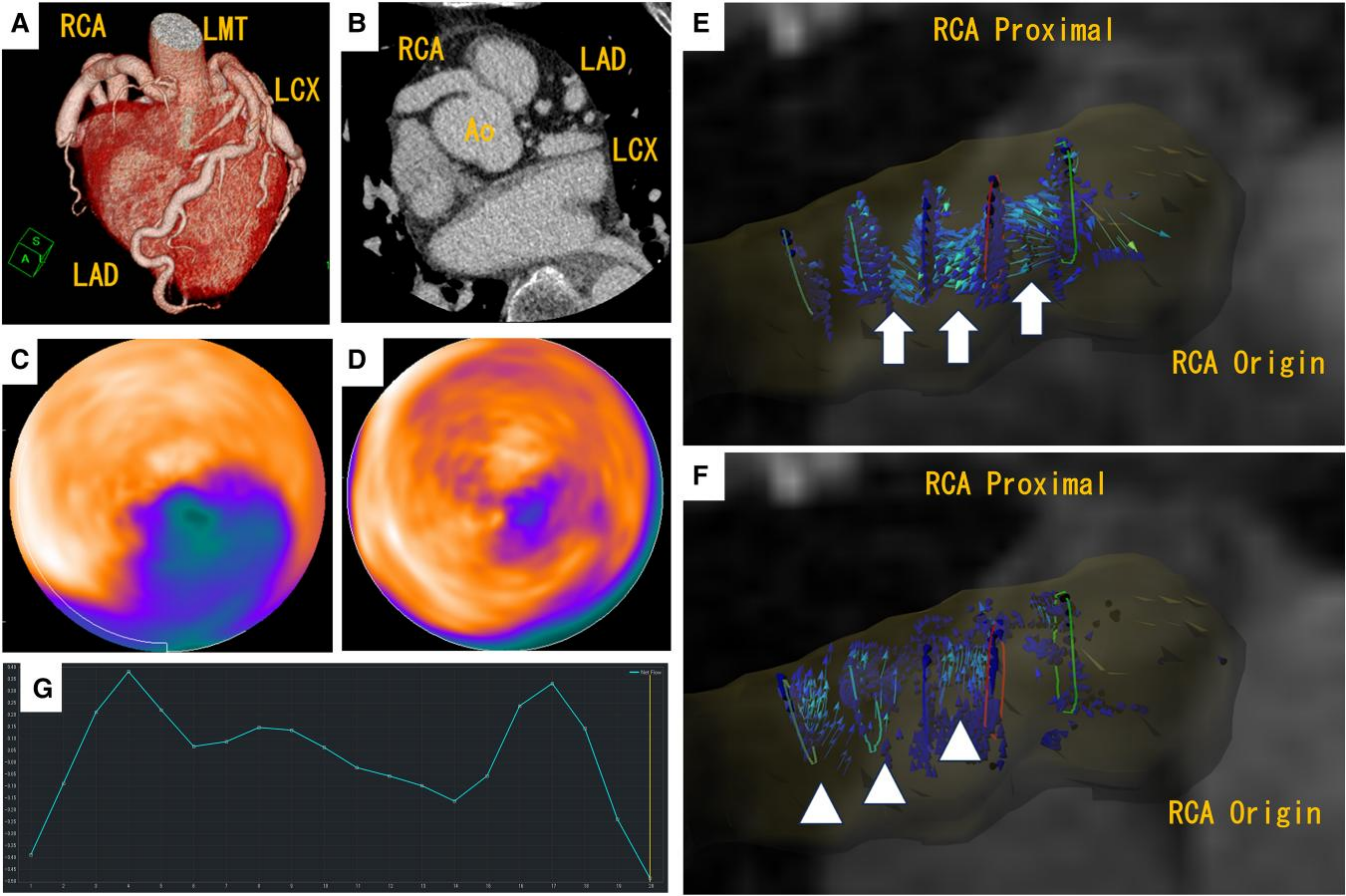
(*A*) and (*B*) Coronary computed tomography angiography. (*A*) Volume rendering imaging and (*B*) axial imaging. Coronary computed tomography angiography revealed coronary artery ectasia in all three coronary arteries without significant stenosis. (*C*) and (*D*) Polar maps from 13N-ammonia positron emission tomography. (*C*) Stress imaging and (*D*) rest imaging. The positron emission tomography images revealed a rest perfusion defect with a larger stress perfusion defect in the inferior and inferoposterior walls, indicating myocardial infarction with concomitant myocardial ischaemia. (*E*) and (*F*) Four-dimensional flow coronary magnetic resonance imaging. These panels demonstrate slow and turbulent flow in the proximal right coronary artery. Forward flow is indicated by a white arrow and backward flow by a white arrowhead. (*G*) Coronary flow velocity waveform. The waveform lacks the typical biphasic pattern with a predominant diastolic phase. Blood flow velocity is generally reduced, the waveform is irregular, and the peak is poorly defined. These findings suggest the presence of abnormal flow, such as slow or turbulent flow.
